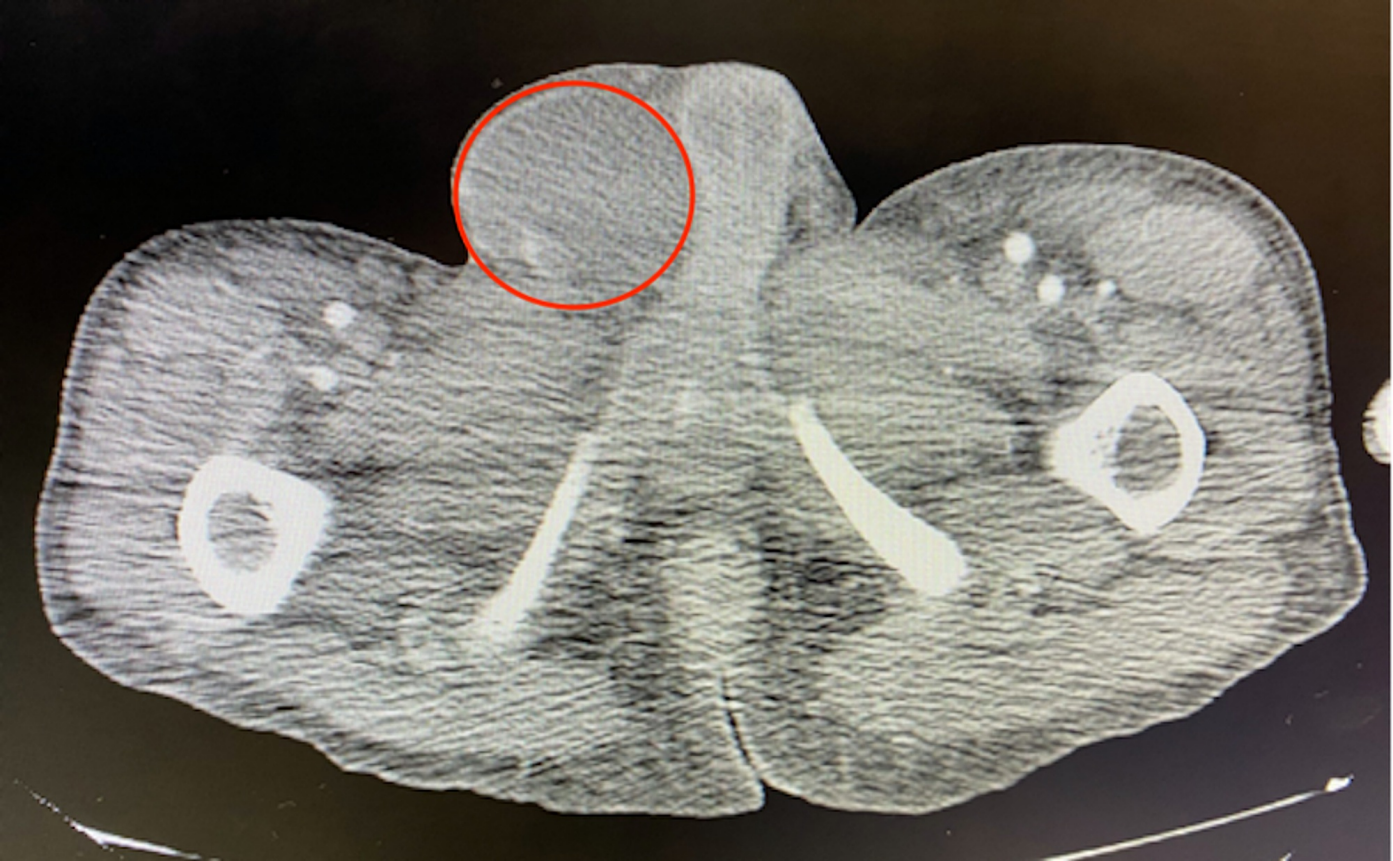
CT scan showing large right testicular mass (red circle) CT, Computed tomography.
